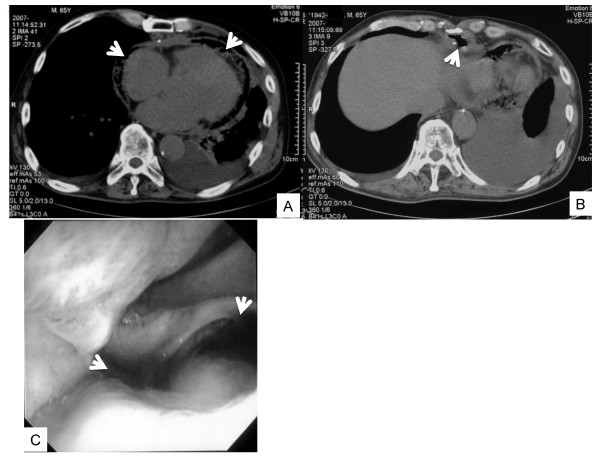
Pre-operative CT scans (A, B): arrows indicate pneumopericardium (A) or gastropericardial fistula (B); Preoperative upper GI endoscope shows the giant open ulcer within gastric tube, indicated by arrows (C).
